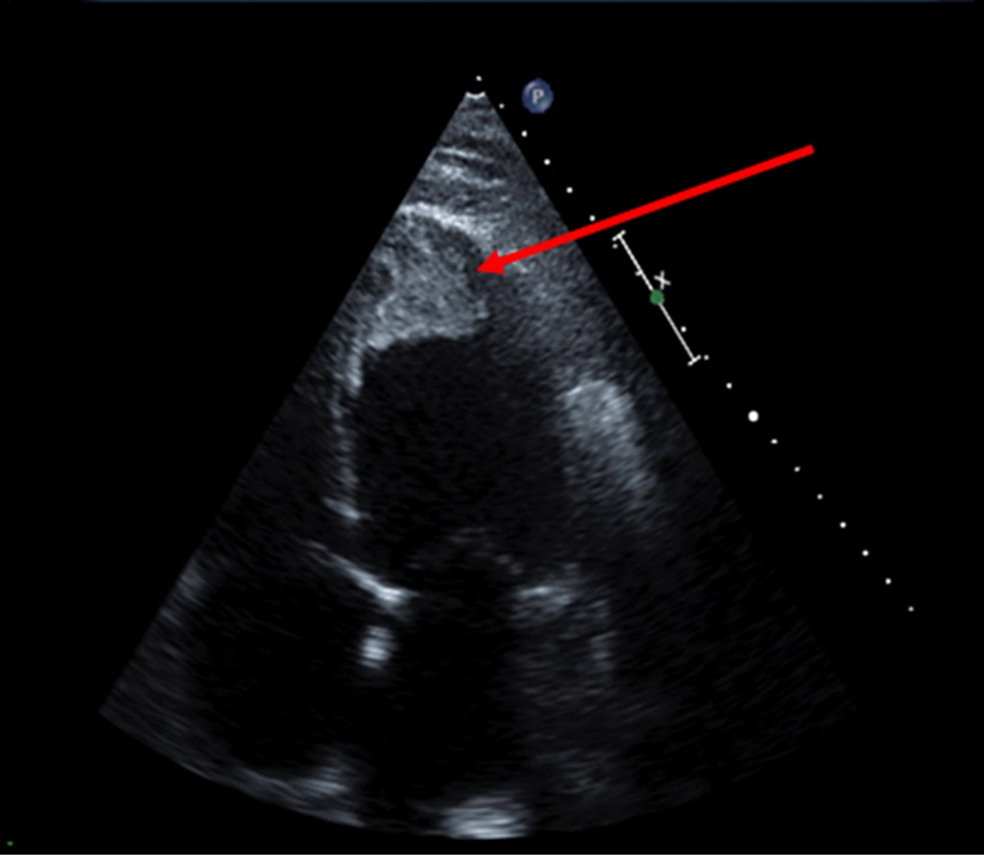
Transthoracic echocardiogram showing apical thrombus in the dilated left ventricle
